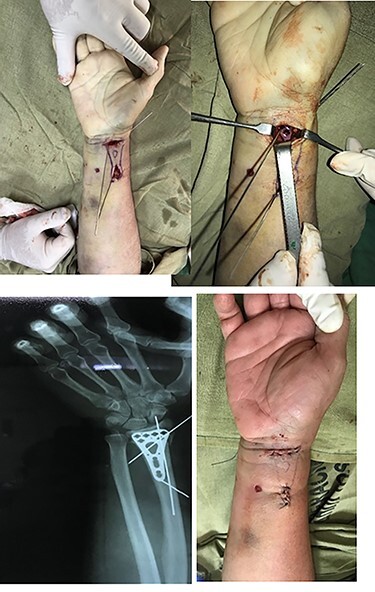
T shape incision. (a) The forearm with the two incisions. (b) Visualizing the plate. (c) X-ray visualizing the correct position of the plate. (d) Both incisions sutured.
